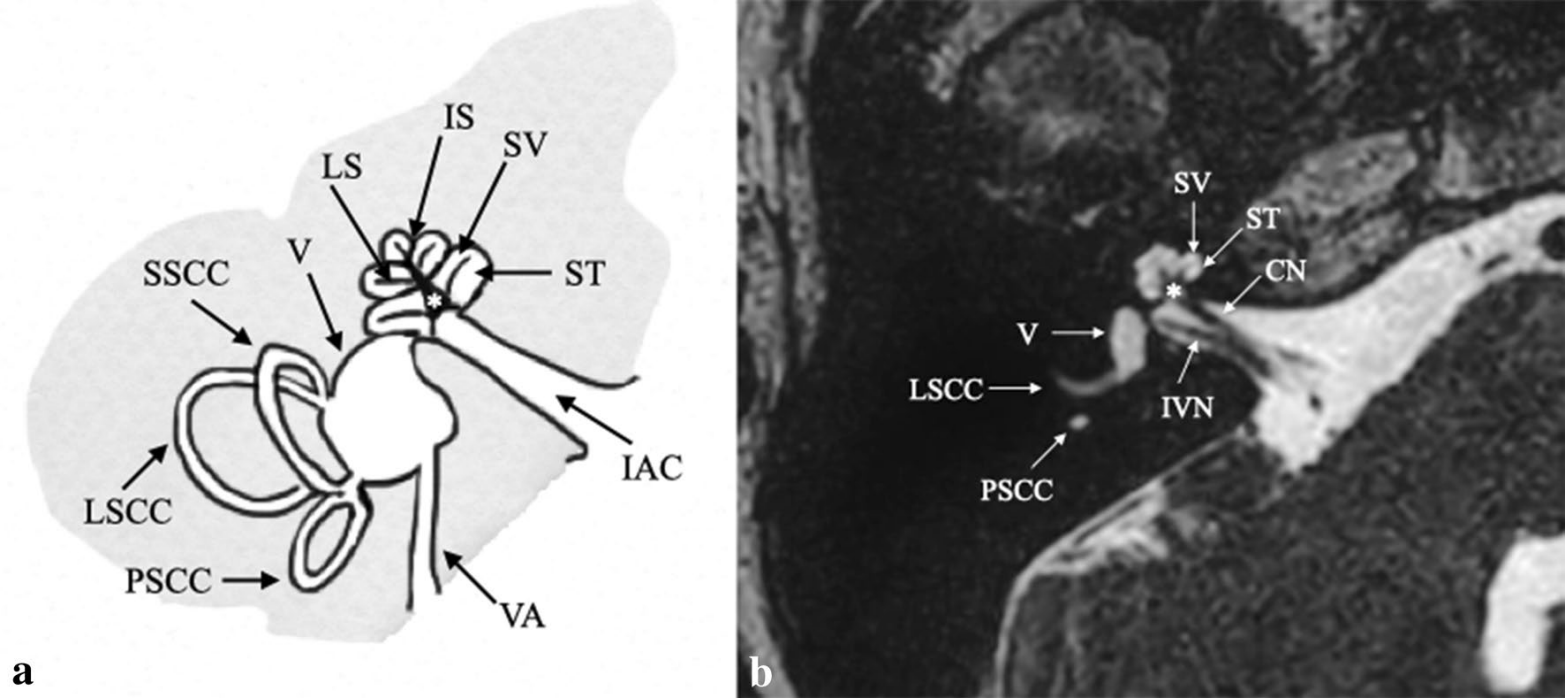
Normal anatomy of Inner Ear structures in drowning (a) and high-resolution 3 T MRI (b) (0.8-mm thick axial MPR from 3D FIESTA sequence). The cochlea consists of a canal that helices 2½ to 2¾ times around a central column of bone (the modiolus, asterisk). The modiolus is the base of the cochlea at the fundus of the internal acoustic canal (IAC). The cochlear spirals are divided by the interscalar septum (IS). A thin osseous spiral lamina (LS) that developments from the modiolus divides the cochlear canal in two compartments: scala vestibuli (SV) and a scala tympani (ST). Legend: CN: cochlear nerve; IVN: inferior vestibular nerve; V: vestibule; LSCC: lateral semicircular canal; PSCC: posterior semicircular canal; SSCC: superior semicircular canal; vestibular aqueduct (VA).
